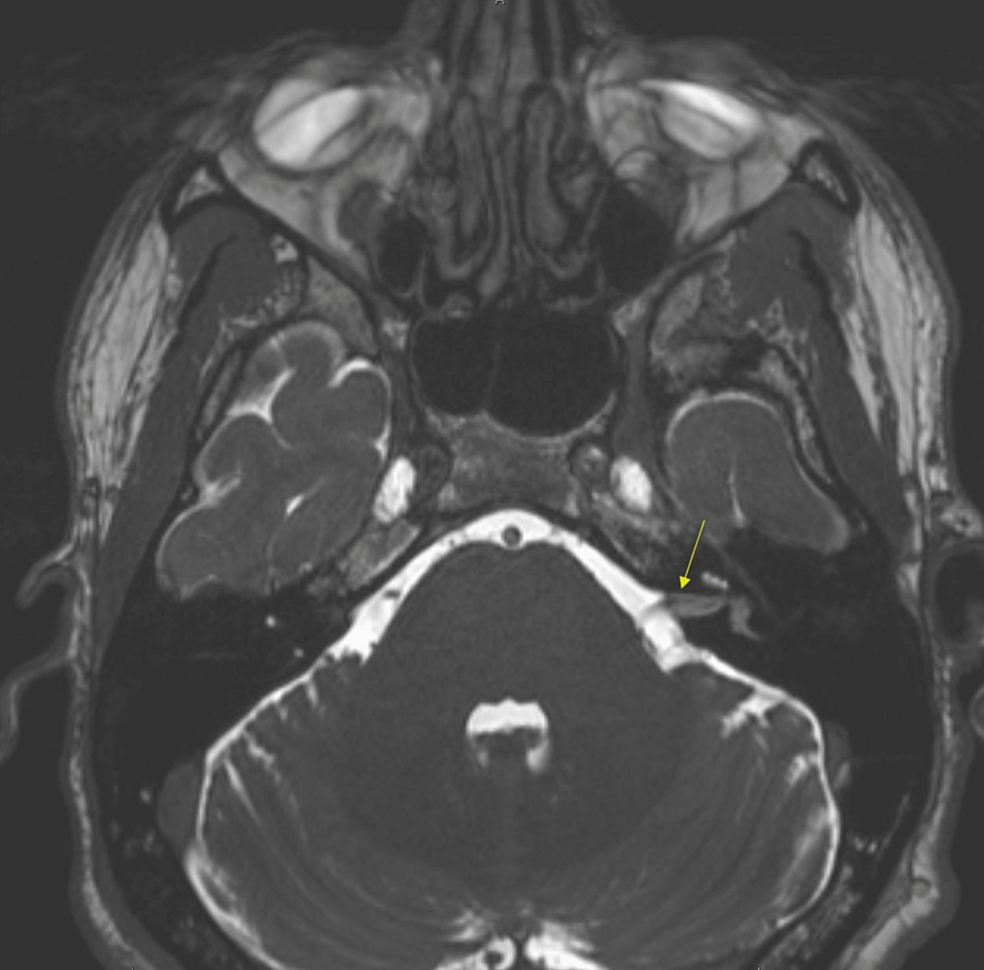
Axial T2 heavy-weighted (CISS) sequence MRI of the brain Seven months after initial imaging and three-month follow-up from the aborted gamma knife procedure. The image shows significantly decreased signal abnormality of the previously seen left IAC lesion (arrow). CISS - Constructive Interference Steady State, IAC - internal auditory canal
